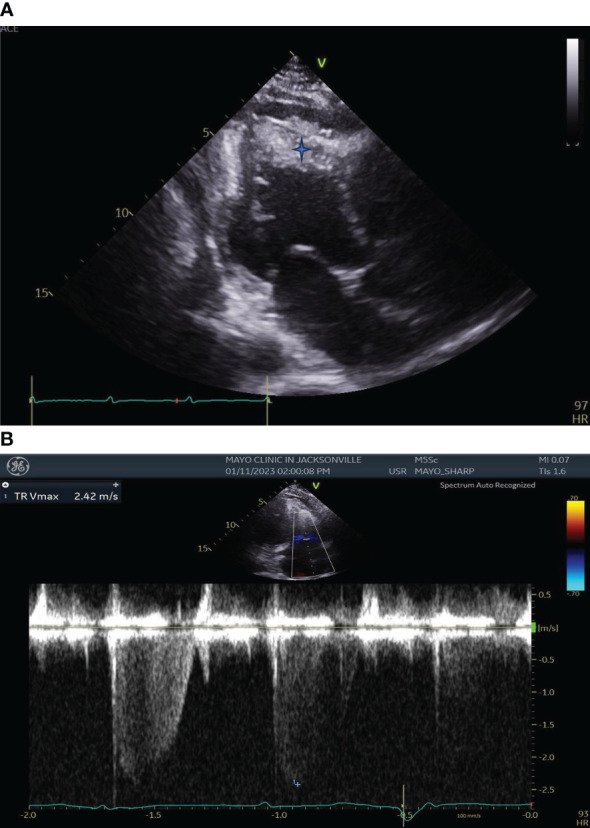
(A) Transthoracic Echocardiography showing left ventricular wall motion abnormalities, enlarged right ventricular chamber size with biventricular apical thrombi (*Blue star)*. (B) Transthoracic Echocardiography showing severe Tricuspid Regurgitation.
